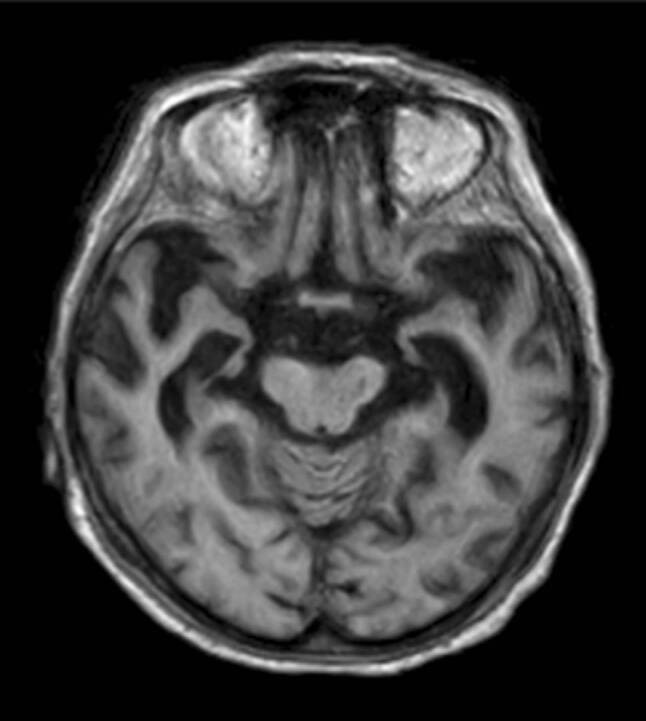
Alzheimer’s disease. A 67-old-patient with Alzheimer’s disease who presented with characteristic bilateral atrophy of the temporal lobe, including the hippocampi, depicted on axial T1-weighted images
